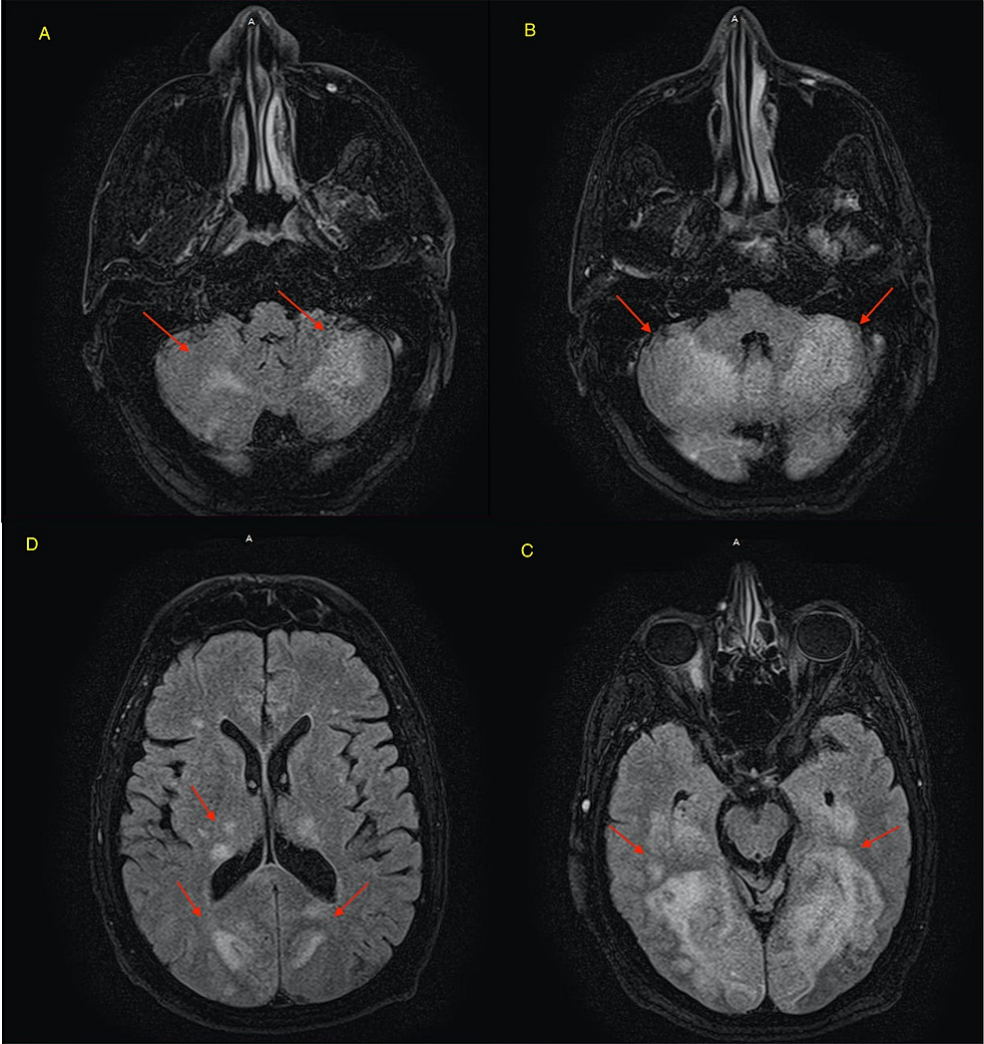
MRI without intravenous contrast on day 7 showed an extensive increased signal intensity in the cerebellum (red arrows in A and B) and in the occipital lobes bilaterally (D and C). There are also abnormal changes in the thalami bilaterally and the splenium of the corpus callosum including a portion of the medial posterior temporal lobes bilaterally. There is associated increased diffusion signal intensity with variable low diffusion signal. These findings are in a posterior circulation distribution bilaterally. Findings are consistent with ischemic changes, which, in a patient with the given history of COVID lung infection, is likely related to sequela of infectious or inflammatory process. Findings related to PRES could be considered though much more extensive than usually expected. PRES, posterior reversible encephalopathy syndrome
